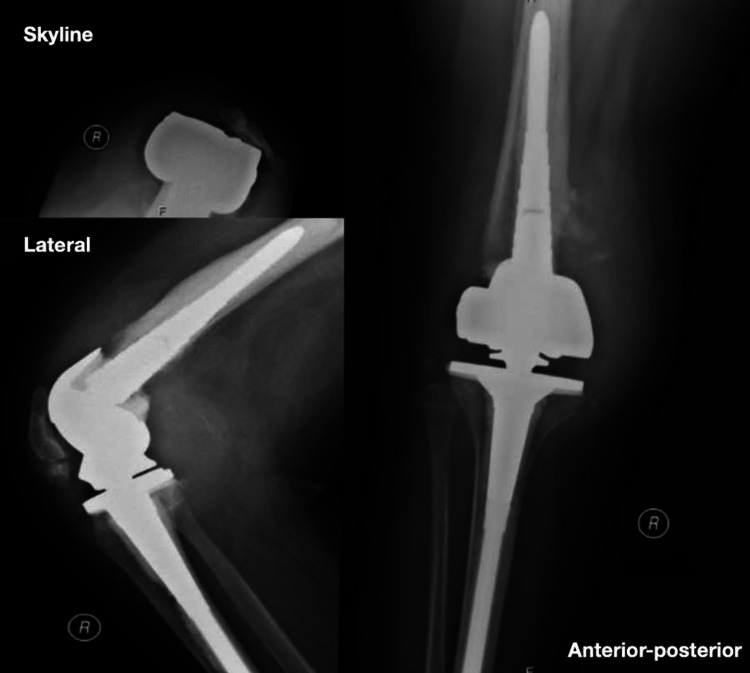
Post-operative X-rays showing the hinged implant that had been used.
